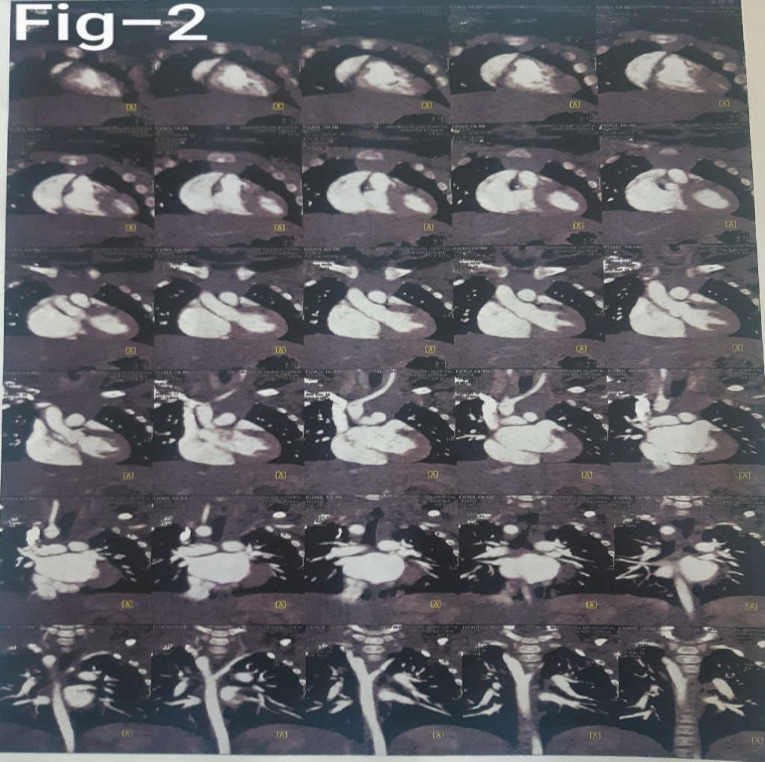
Multi-slice spiral computerized tomography (CT) angiography; Right-sided aortic arch with an aberrant left subclavian artery with notable compression on esophagus and prominent main pulmonary artery and its branches due to left to right shunt
